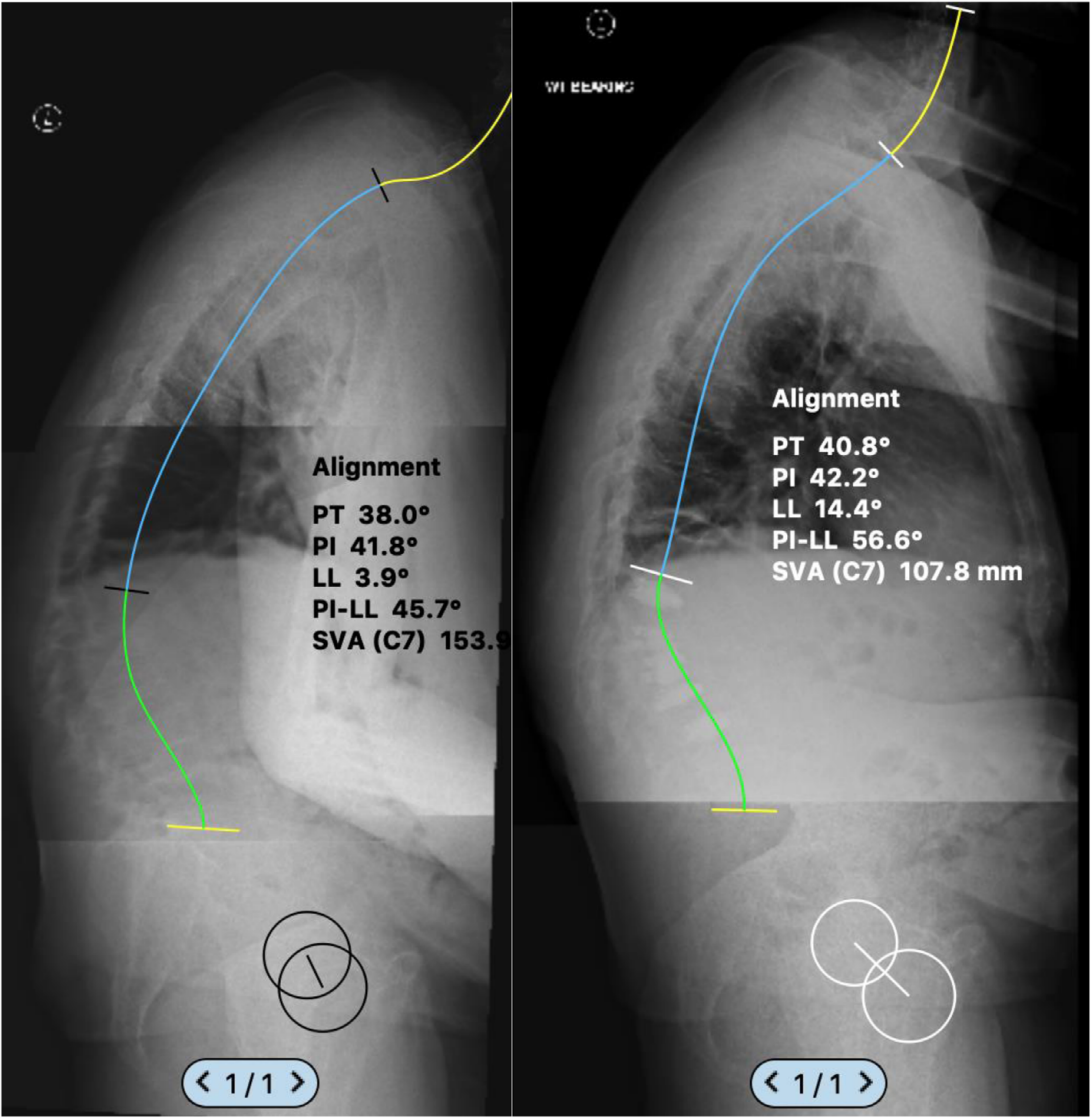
Pre and 2 years postoperative lateral whole spine radiographs showing improvement in sagittal balance after augmentation (T12-L4) with the Osseofix™ implant. using Surgimap™ software (PT = pelvic tilt, PI = pelvic incidence, LL = Lumbar lordosis, SVA = sagittal vertical axis).
